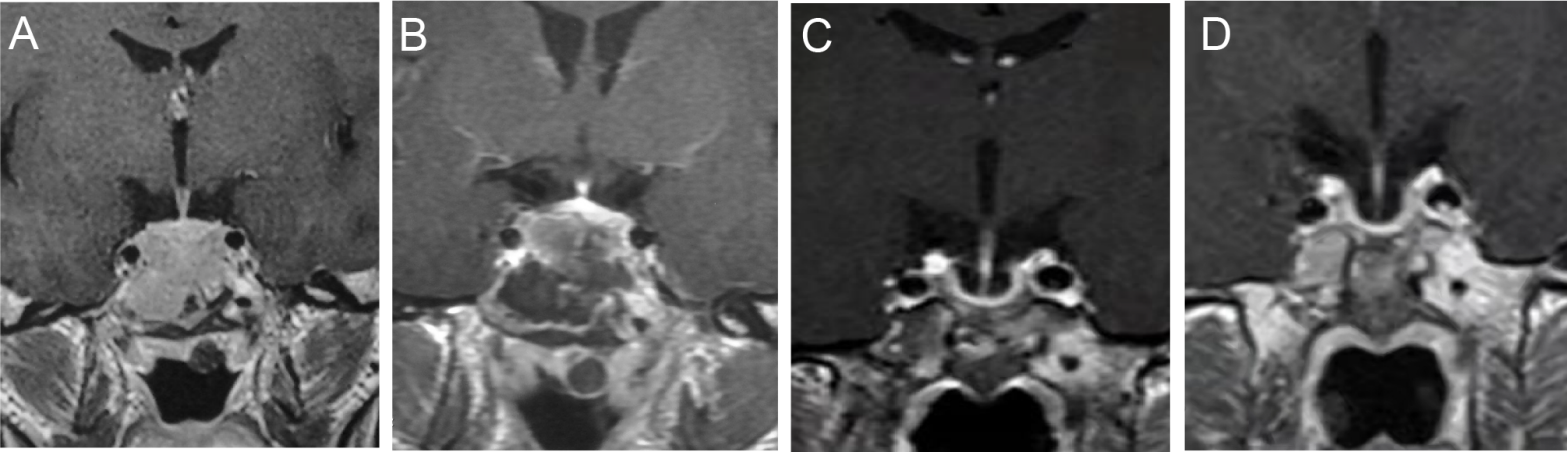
T1WI by contrast enhanced MRI before, after the surgery and in the follow up. MRI before the surgery (A). One week after the surgery (B). Four months before HCQ and CAB combination treatment (C). Three months after HCQ and CAB combination treatment (D).
